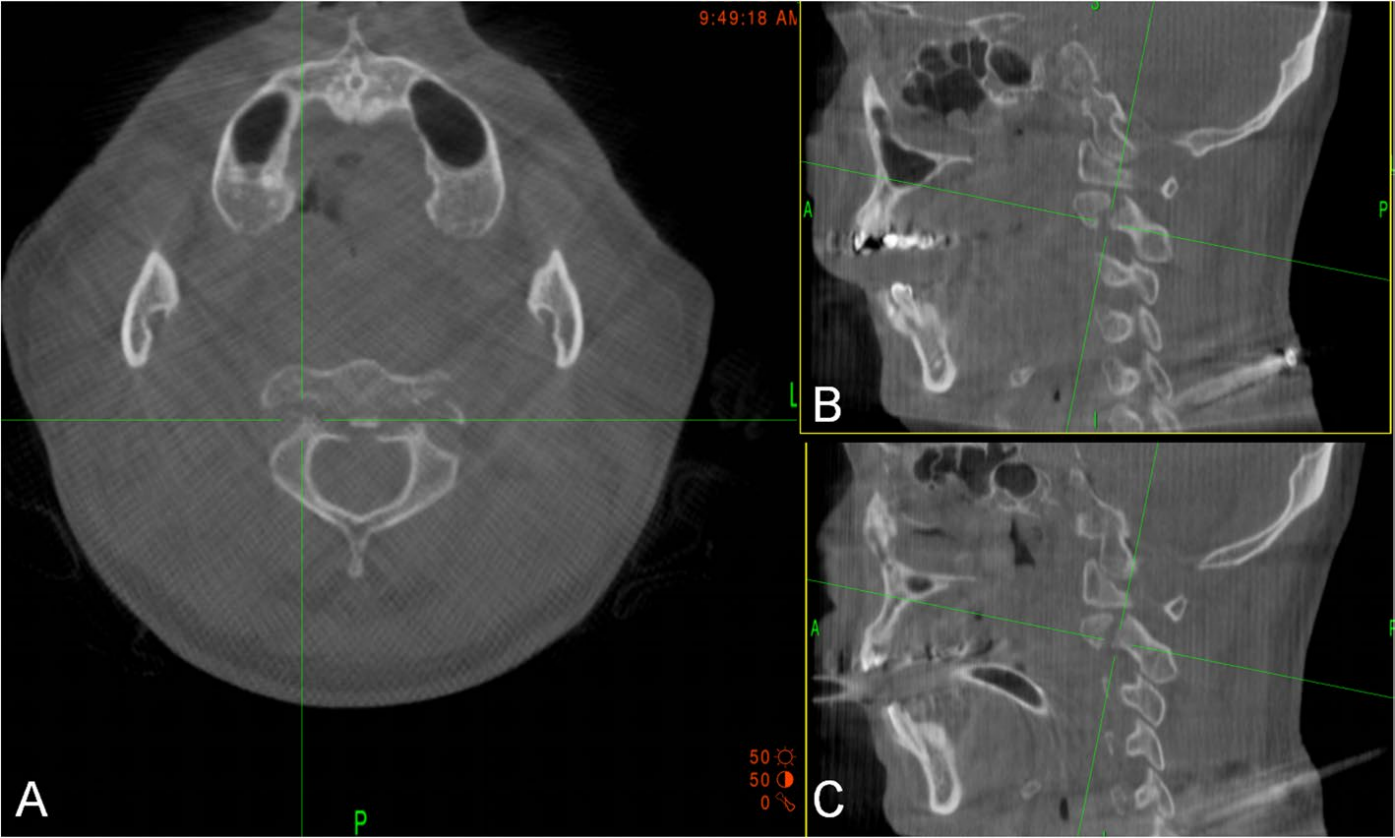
Intraoperative CT scan was automatically registered to the image guidance system, which showing fracture of pars on both the sides on parasagittal sections (A, B, C)
